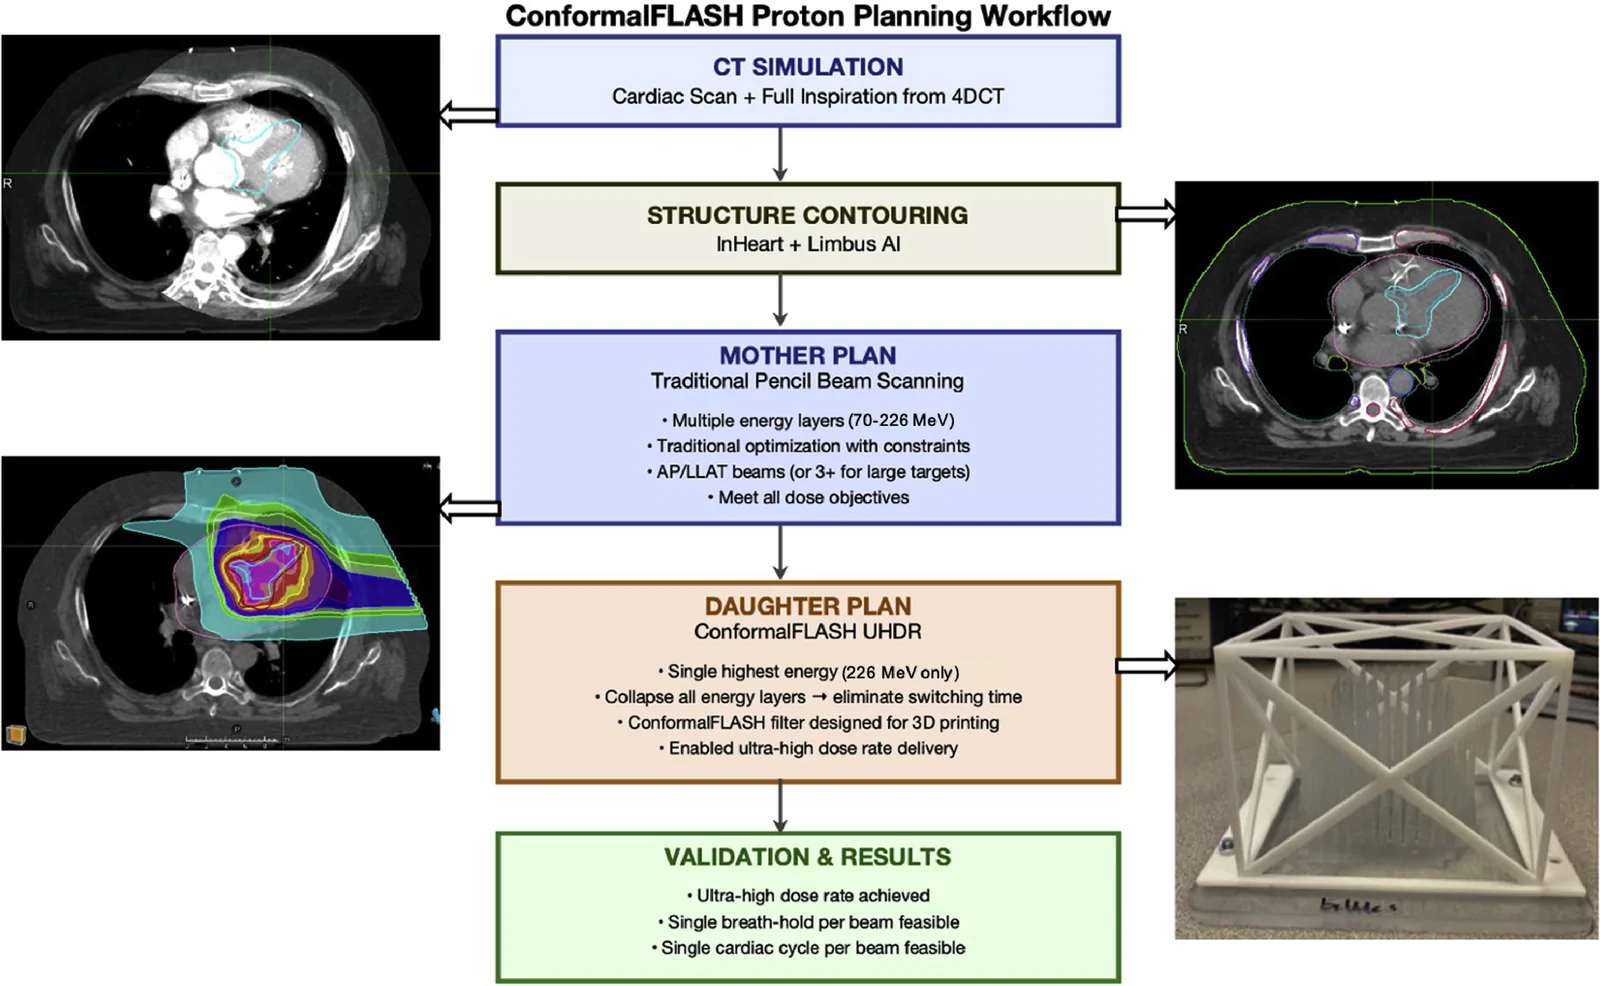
Creation of a ConformalFLASH proton daughter plan for cardiac radioablation cases. *Abbreviations:* AP/LLAT = anterior-posterior and left lateral; CT = computed tomography; UHDR = ultra-high-dose-rate.
